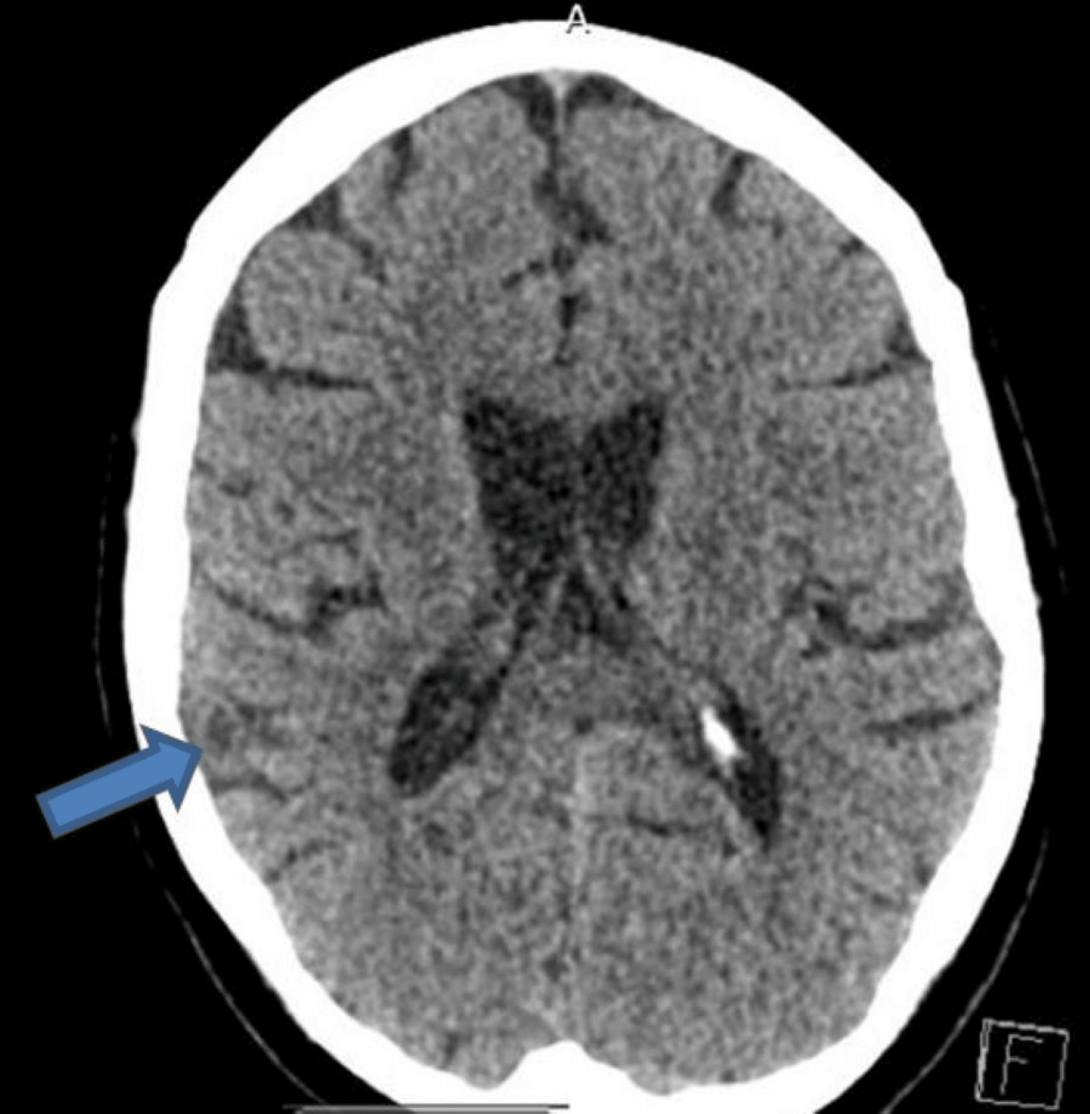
Head CT (axial view) showing right parietal lobe hypoattenuation (arrow)
